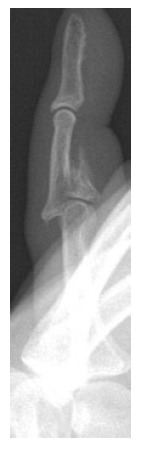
Patient 3 lateral preoperative radiograph.
